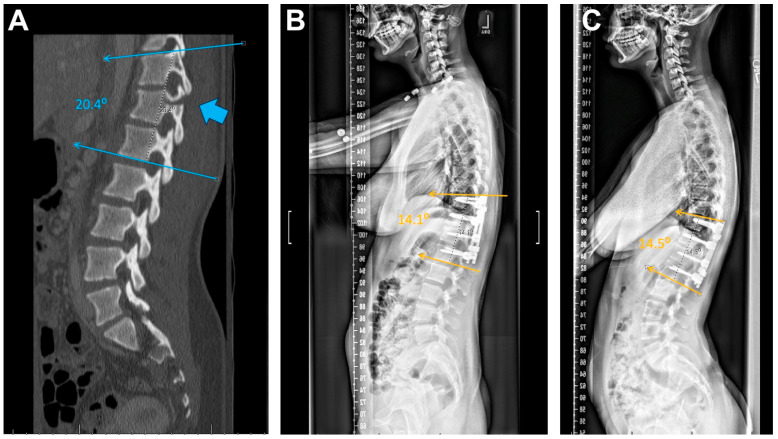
Example spinal alignment assessment in a patient managed with open screw fixation. (A) CT-thoracolumbar spine with parasagittal view demonstrating fracture morphology (arrow) at T12. Standing X-rays with lateral views at post-operative day 1 (B), and >2-year (C).
